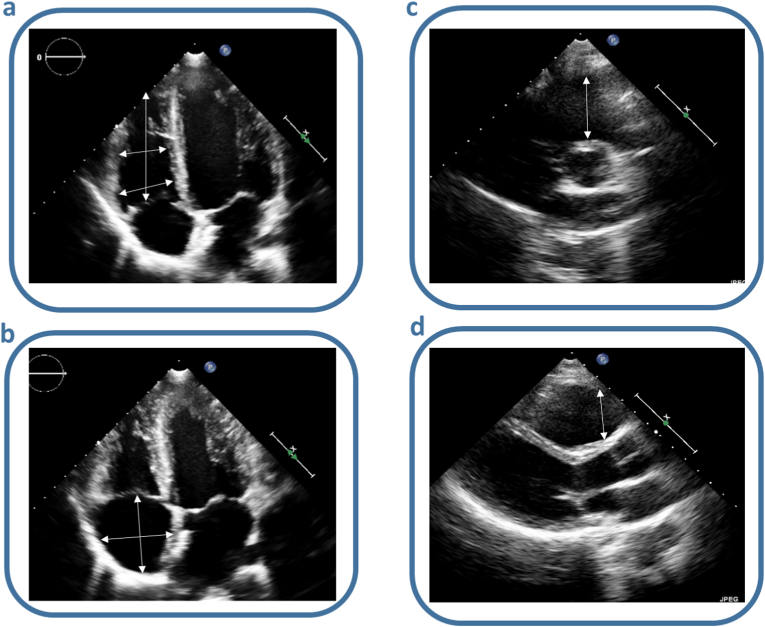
Overview of the methodology used for RV dimensions measurements: in the apical 4-chamber view, the end diastolic basal, mid a longitudinal RV a), end-systolic major and minor RA b) and the end-diastolic proximal right ventricular outflow tract on the parasternal short c) and long axis d).
